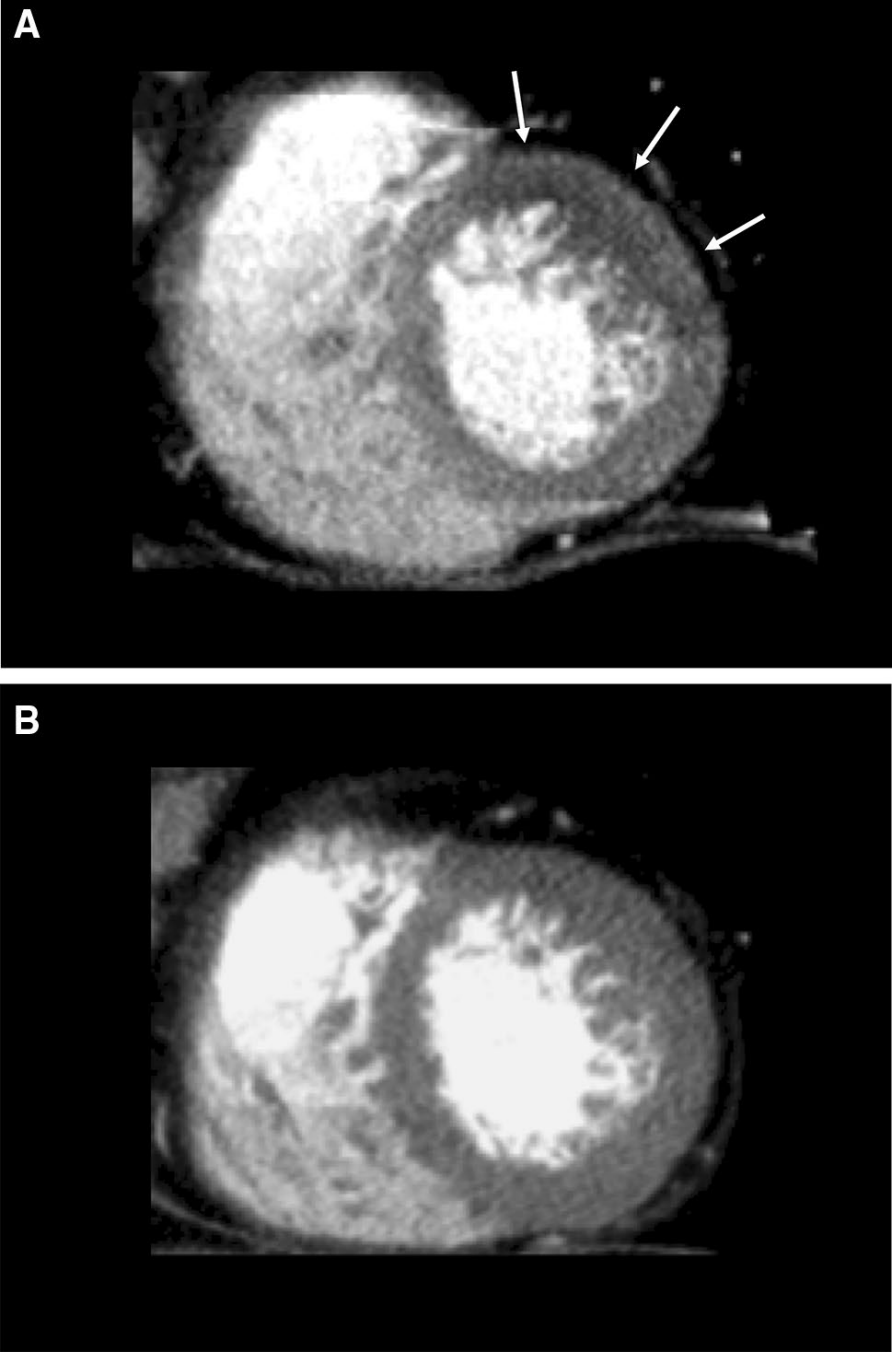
Static CTP scans for single-vessel disease in a 68-year-old woman with chest pain. She had hypertension and dyslipidemia. a A short-axis CTP image following stress shows a subendocardial perfusion defect in the mid anterolateral wall (arrows). b A rest CTP image in the same position as a shows no perfusion defect, consistent with myocardial ischemia. *CTP* computed tomography perfusion
